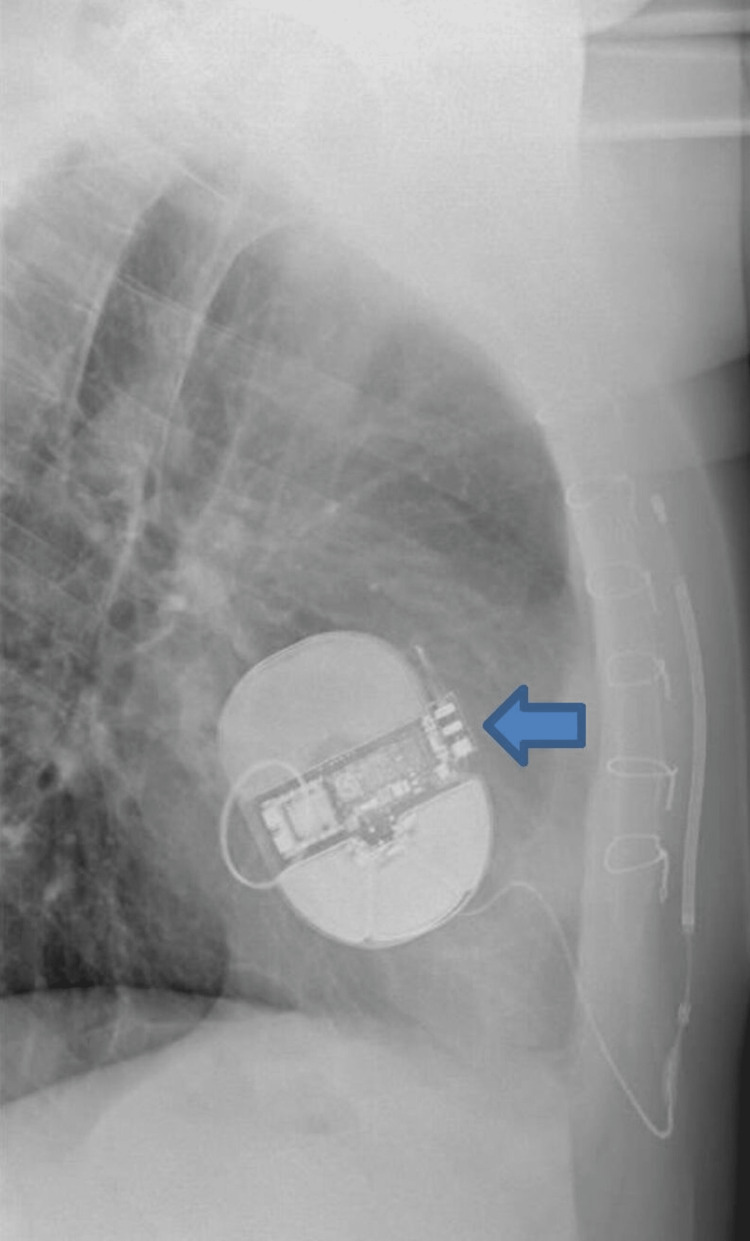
Chest radiography lateral view showing the ICD (pointed arrow). ICD = implantable cardioverter defibrillator
